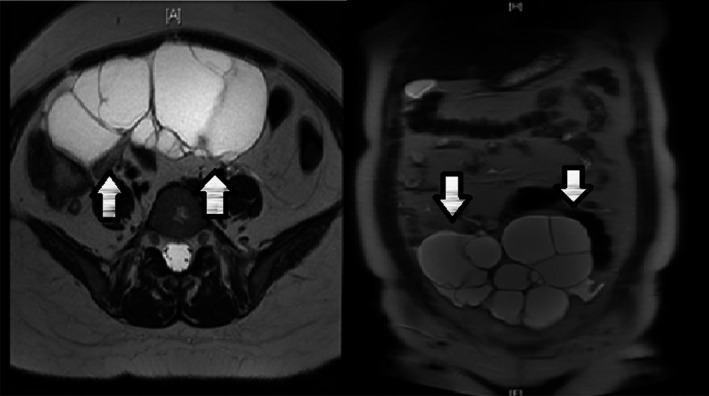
Magnetic resonance imaging (MRI) findings were consistent with bilateral multiloculated cystic ovarian lesions. The right side measures 13.6 × 15.4 × 6.8 cm, and the left side measures 3.6 × 7.4 × 3.1 cm in the anteroposterior, transverse, and craniocaudal dimensions.
